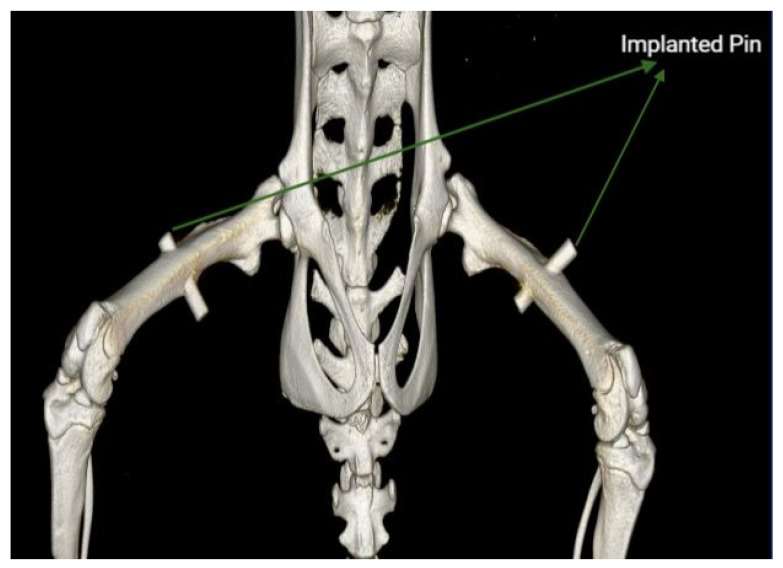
CT image showing the Mg implant positioning on day 7. Acquired with an IRIS PET/CT scanner (Inviscan Imaging System, Strasbourg, France), visualized in an OsiriX DICOM Viewer (Pixmeo, Switzerland).
